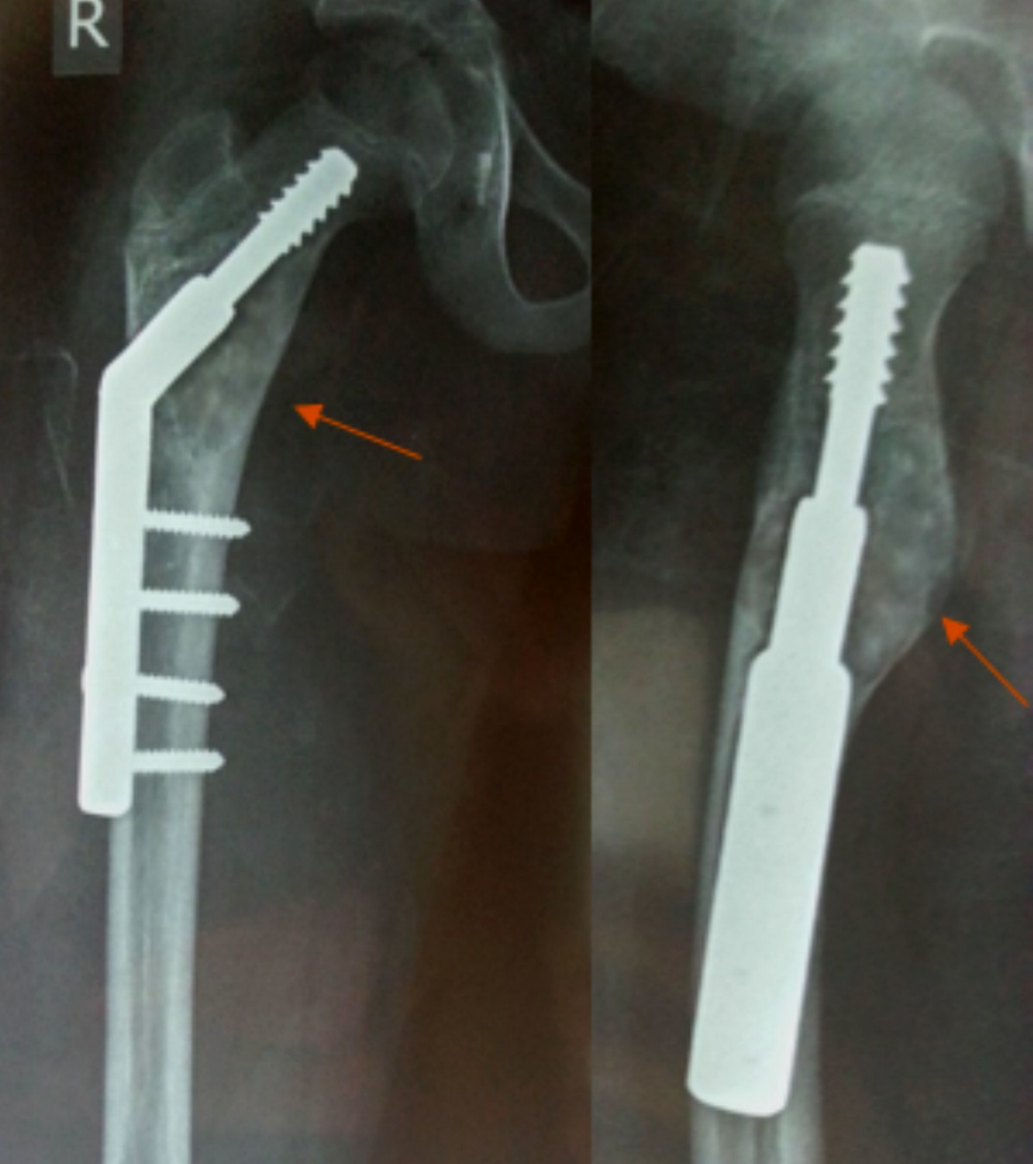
Postoperative radiograph (anteroposterior and lateral views) Shows curettage of the lesion with bone grafting and fixation with dynamic hip screw (DHS).
